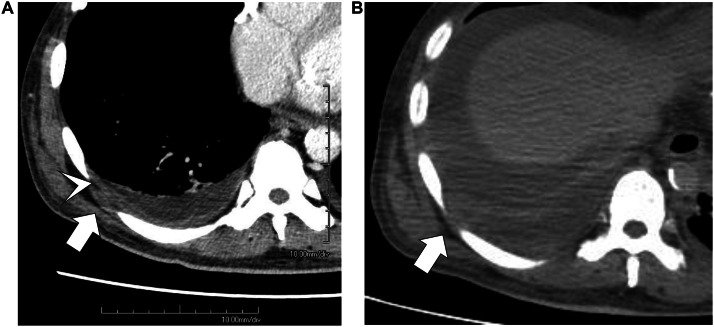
Right pleural space on contrast-enhanced CT at admission (A) and plain CT at death (B). Empyema with septal structures is observed in the right lung. The right intercostal muscles are partially displaced laterally (arrows), reflecting the formation of an extrapleural abscess. On contrast-enhanced CT, extrapleural fat tissue appears hypodense but no proliferation is noted (arrow head). Its structure also appears indistinct.
